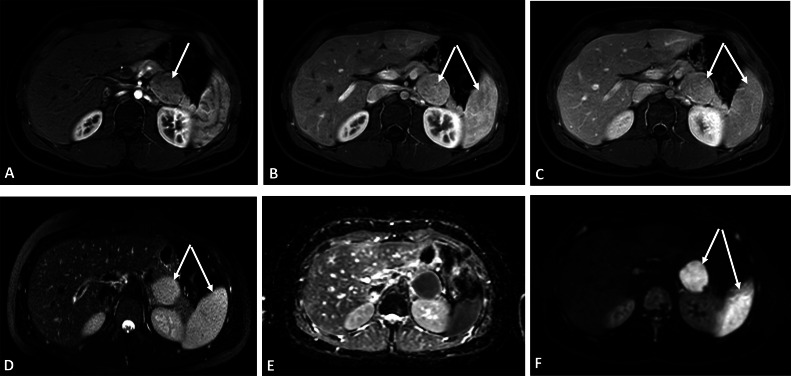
Abdominal MRI with IV contrast demonstrated heterogeneous typical enhancement of the spleen in T1-weighted axial contrast-enhanced early arterial phase images (A), with similar enhancement observed in the retroperitoneal mass(arrow). Late arterial and portal phases also showed enhancement of the lesion comparable to the spleen (B, C). The lesion appeared as a hypersignal mass in T2-weighted images (arrow) (D). In the ADC (Apparent diffusion coefficient) map and DWI (Diffusion-weighted imaging) sequences (E, F), the lesion showed a high diffusion signal similar to the spleen.
